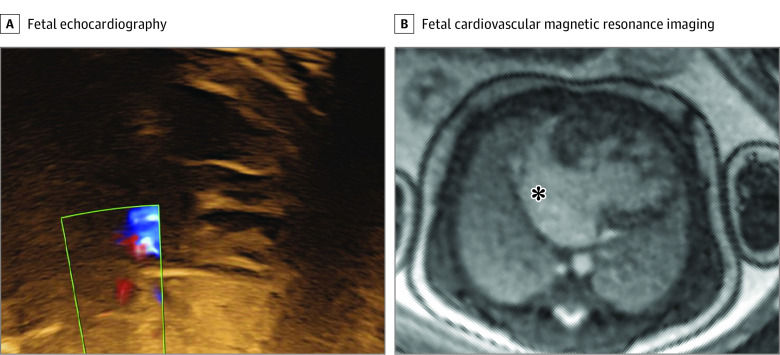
Evaluation of Atrial Restriction in a Fetus With Hypoplastic Left Heart Syndrome Fetal echocardiography could not visualize the atrial cavity or Doppler flow across the atrial septum due to poor acoustic windows (A). Fetal cardiovascular magnetic resonance showed a large interatrial communication, indicated by the asterisk, and no nutmeg pattern (B). Therefore, the risk of restrictive atrial septum was considered low (albeit a membrane could not be ruled out), and the fetus was planned for a vaginal delivery without cardiac catheterization laboratory on standby. Contrast and brightness in the CMR image have been optimized for printing (case 5) (eTable in the Supplement).
